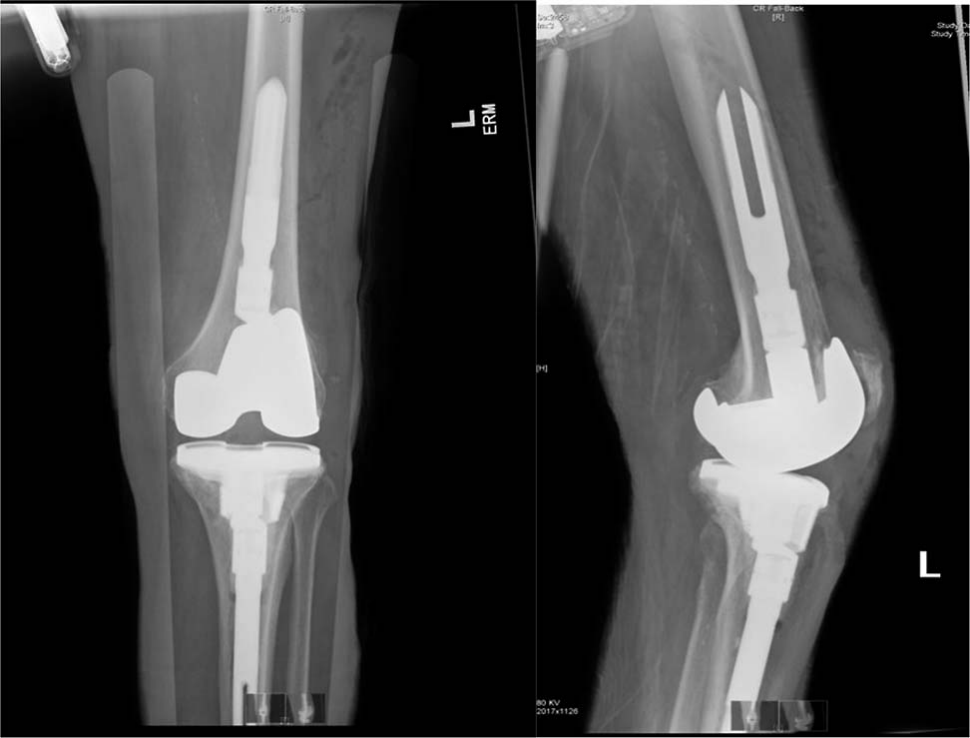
Radiographs demonstrating replacement of the long tibial and femoral stems after stage 2 of the revision. Alignment appears excellent. Air in the soft tissues is noted as expected.
